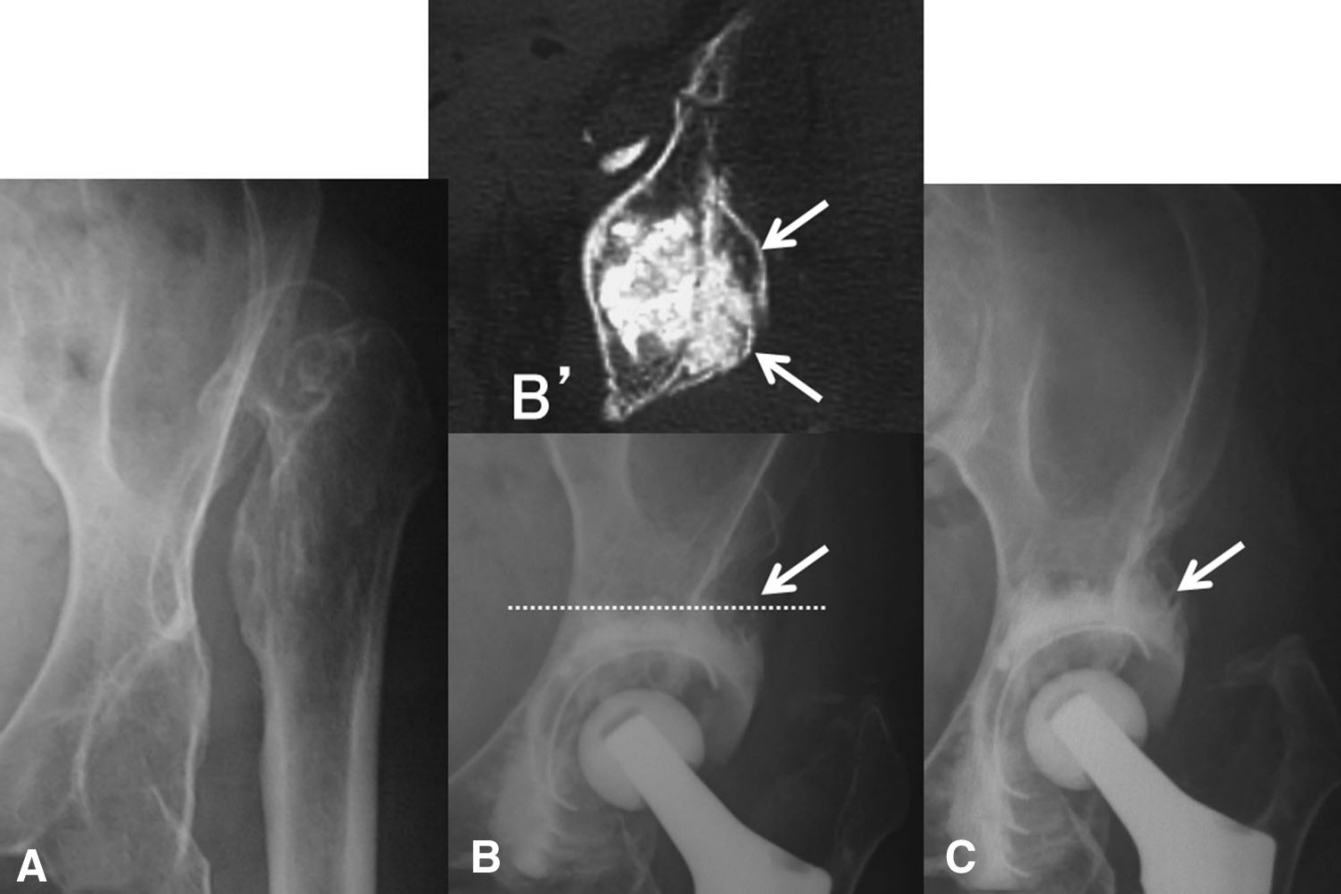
Dislocation case (left hip) with shallow acetabulum. Remodeling and reorientation of the additional bulk bone grafting (arrow) with morselized bone chips plus hydroxyapatite granules are shown in consecutive radiographs of a left hip preoperatively (A), 3 weeks (B) with CT scanned at the dotted line (B′), and 6 years (C) postoperatively of a 70-year-old woman at the time of surgery.
